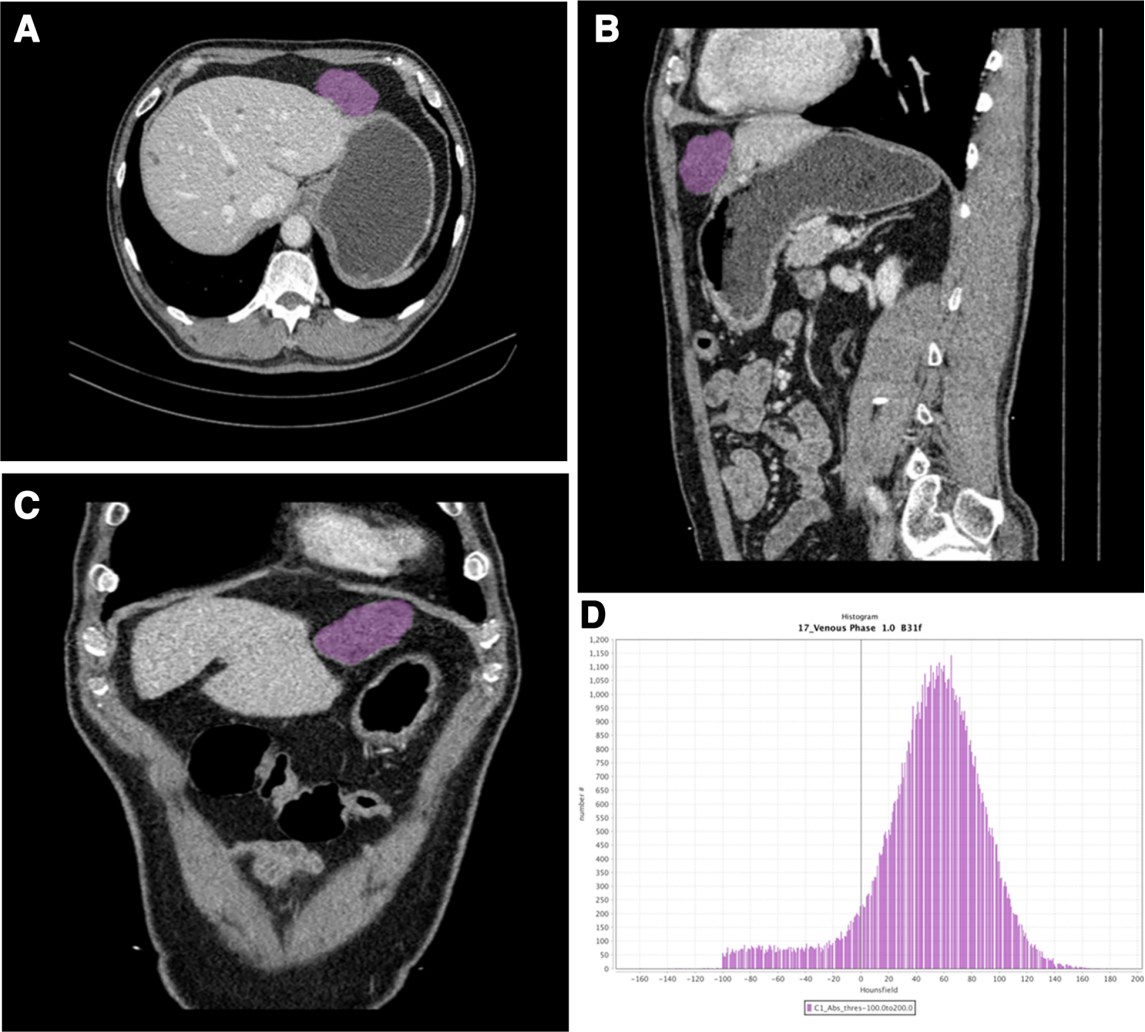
Abdominal portal venous phase CT images of a 33-years-old woman. Texture features were extracted from the primary tumor area (purple overlay). a transverse section, b median sagittal section, c coronal section, d Histogram
